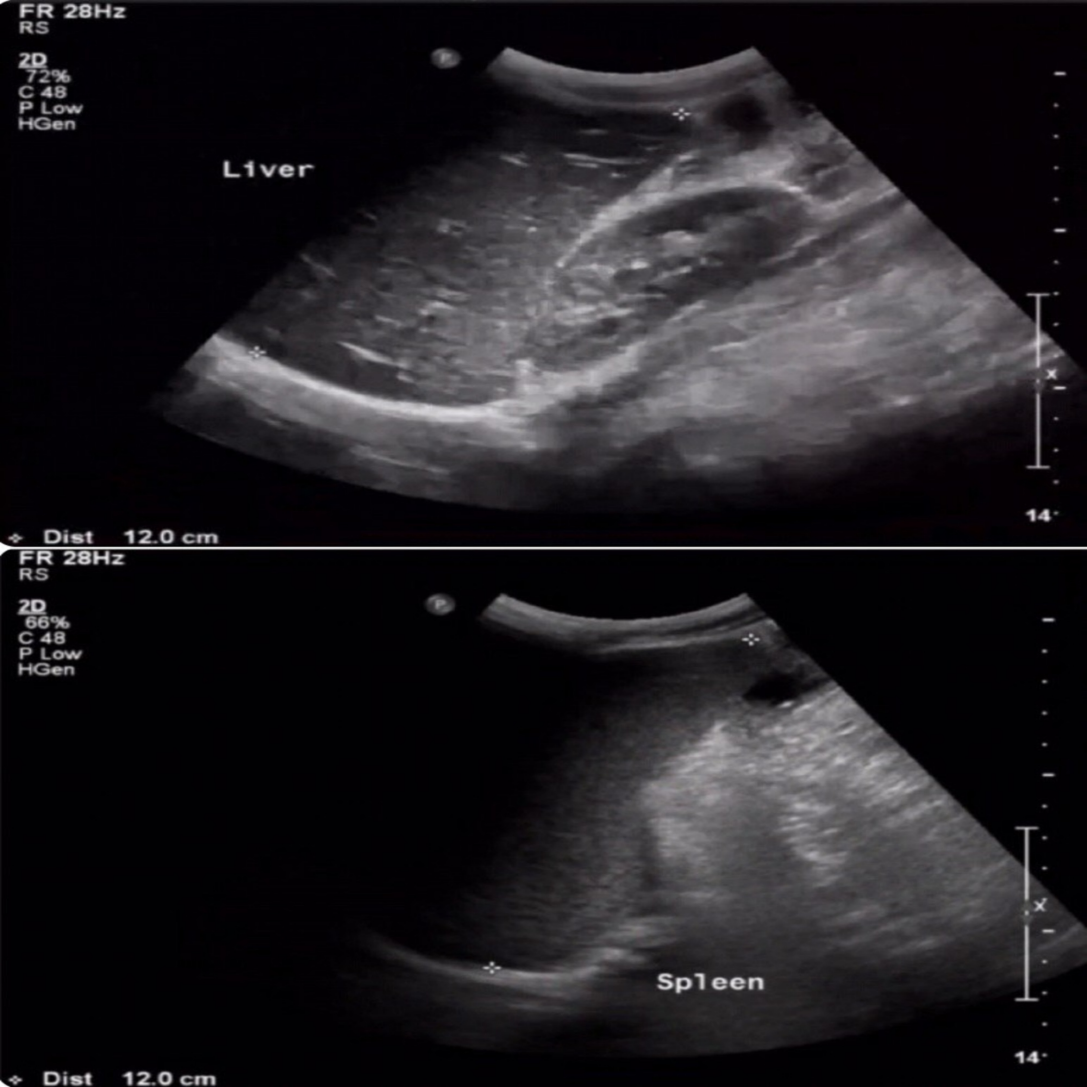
Abdominal ultrasound showing hepatosplenomegaly
